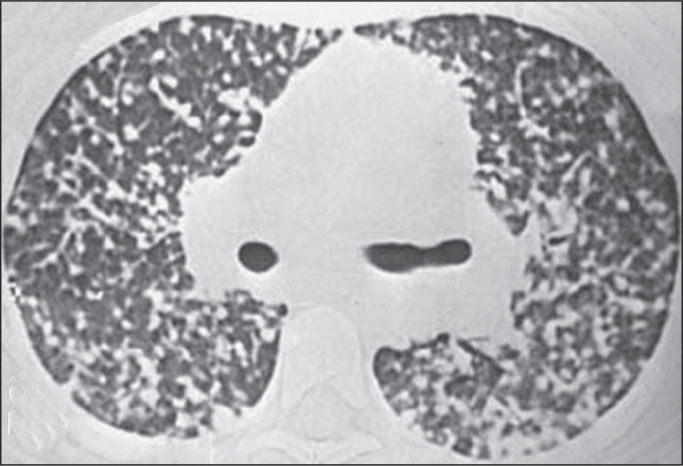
CT of a 6-year-old with positive serology, showing small nodules
